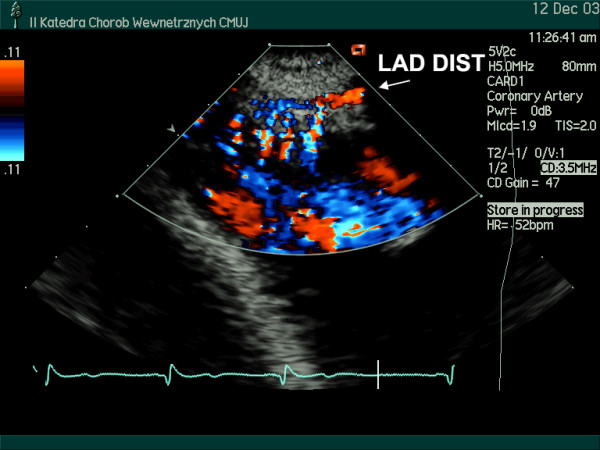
Modified apical 2-chamber view, apical area, color Doppler examination. The LAD and the penetrating intramyocardial arteries (vertical perforators branching of the LAD) are seen.
